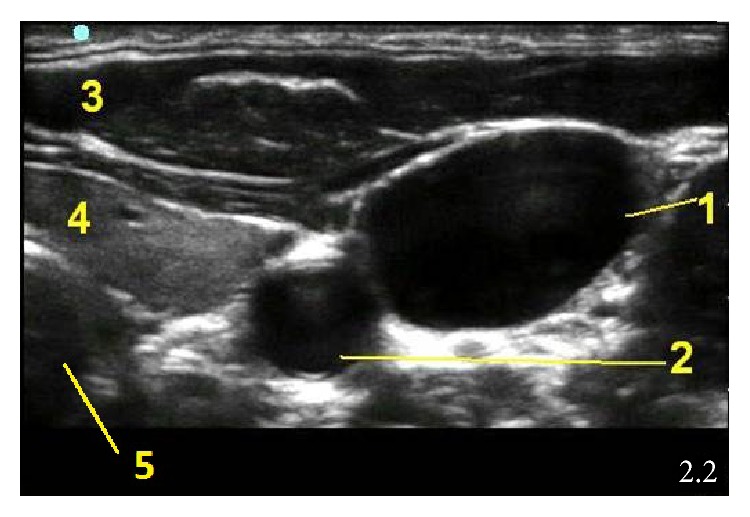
Transverse neck ultrasound image at the level of C6 vertebra. (1) Internal jugular vein, (2) common carotid artery, (3) subcutaneous tissue, (4) thyroid gland, and (5) trachea.
